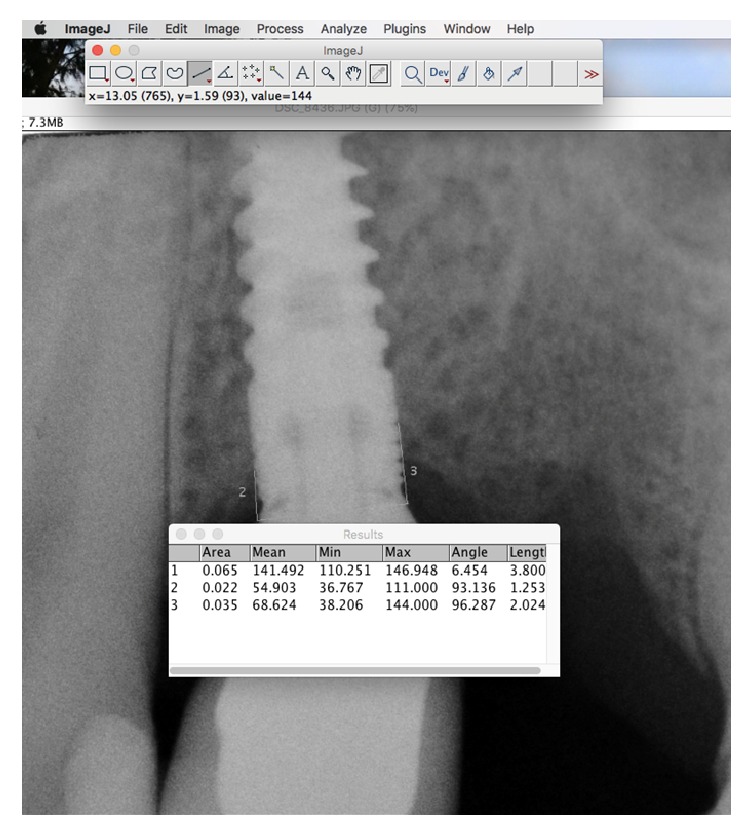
A computer-assisted calibration was performed and linear measurements of MBL were Also it was measured the known implants length and diameter to guarantee a correct measurement even if the implant was slightly angulated on the radiograph.
